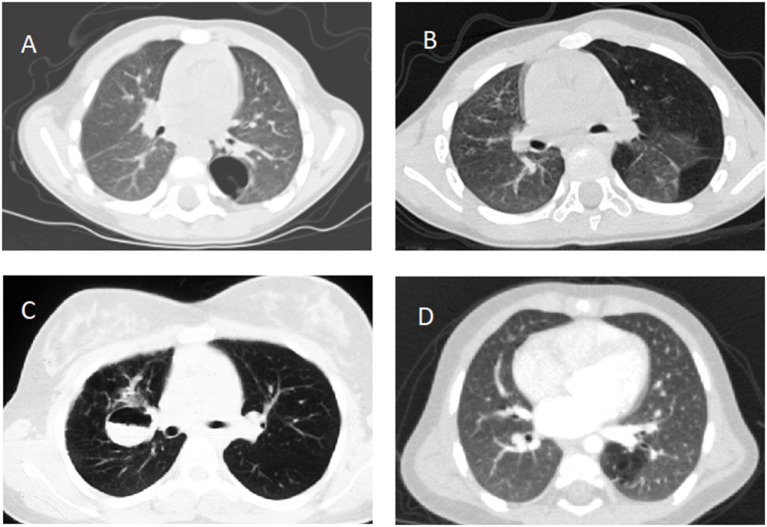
Computed tomography scans documenting (A) type 1 congenital pulmonary airway malformation in the left lower lobe, (B) congenital lobar emphysema involving the left lung, (C) fluid filled bronchogenic cyst in the right lung, (D) intralobar pulmonary sequestration in the left lower lobe, all confirmed after lobectomy except (B).
